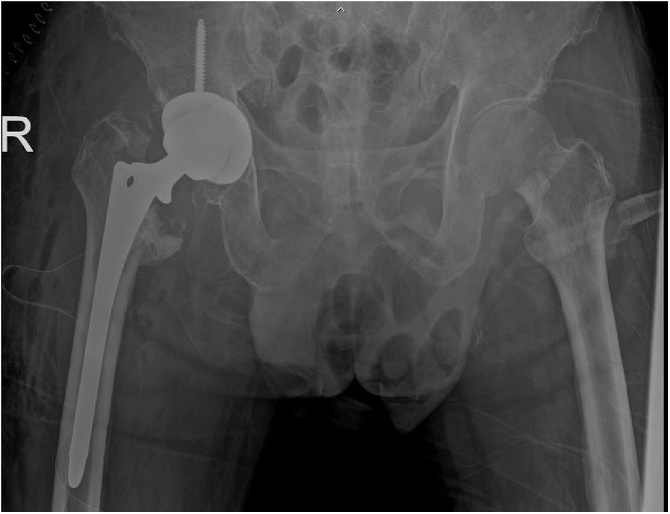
Immediate post-operative plain radiographs after revision total hip replacement.
